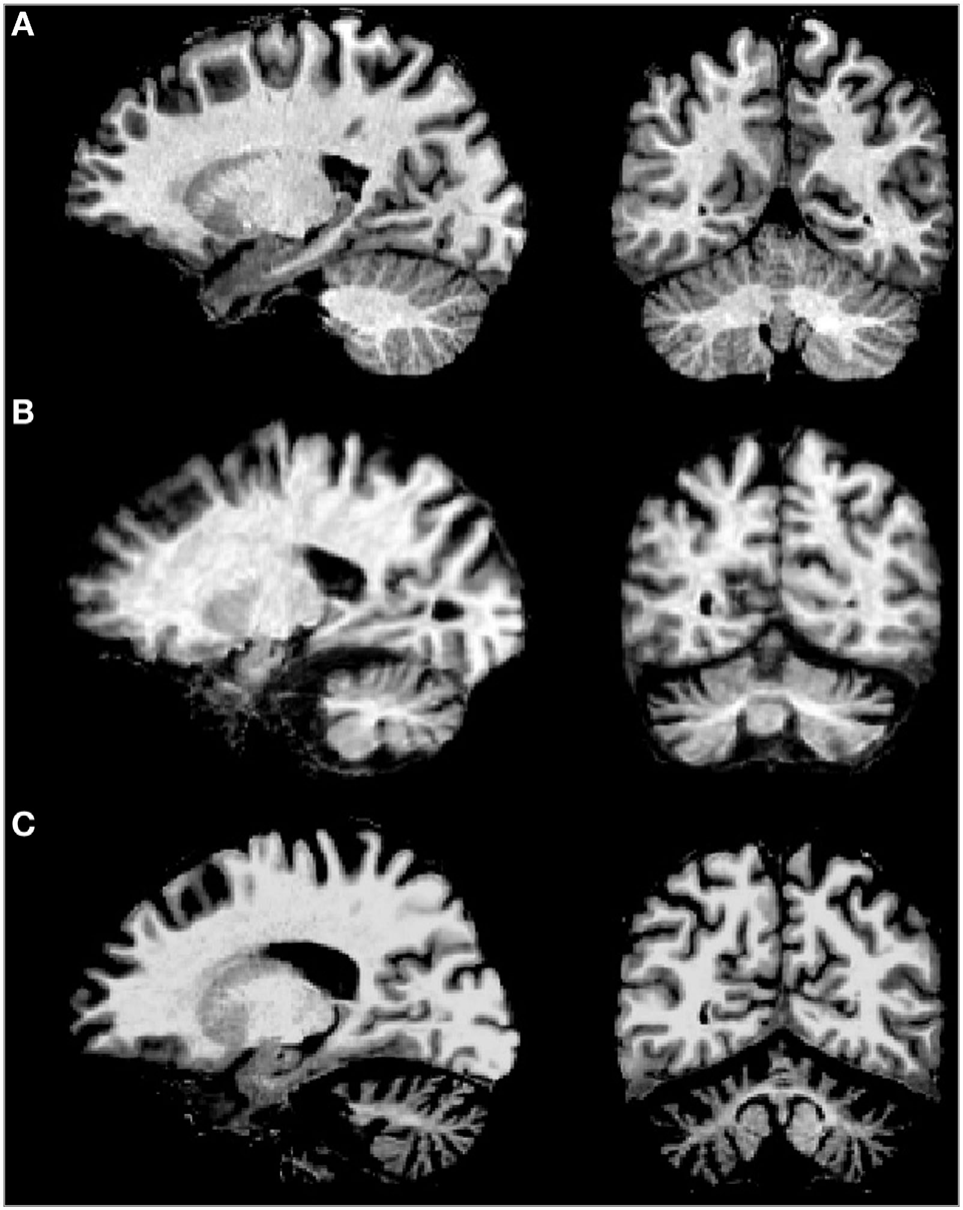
MRI of sagital (left) and coronal (right) examples of (A) normal brain, (B) spinocerebellar ataxia type 2 (SCA2) patient with early clinical manifestation, and (C) SCA2 patient with full ataxia manifestation. Note the severe cerebellar volume loss in gray and white matter in advanced stages of the disease.
